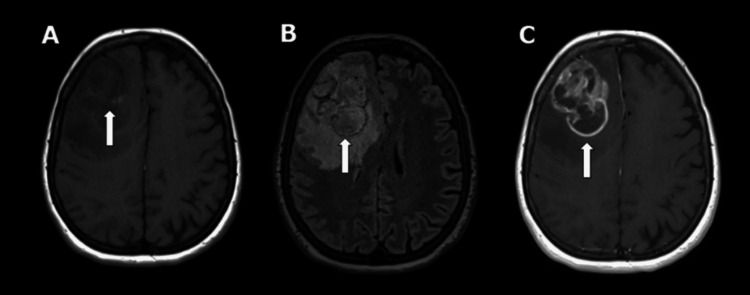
Axial MRI images showing a large right frontal mass in T1 (A) with perifocal edema in FLAIR (B) and contrast enhancement (C) FLAIR: fluid-attenuated inversion recovery
